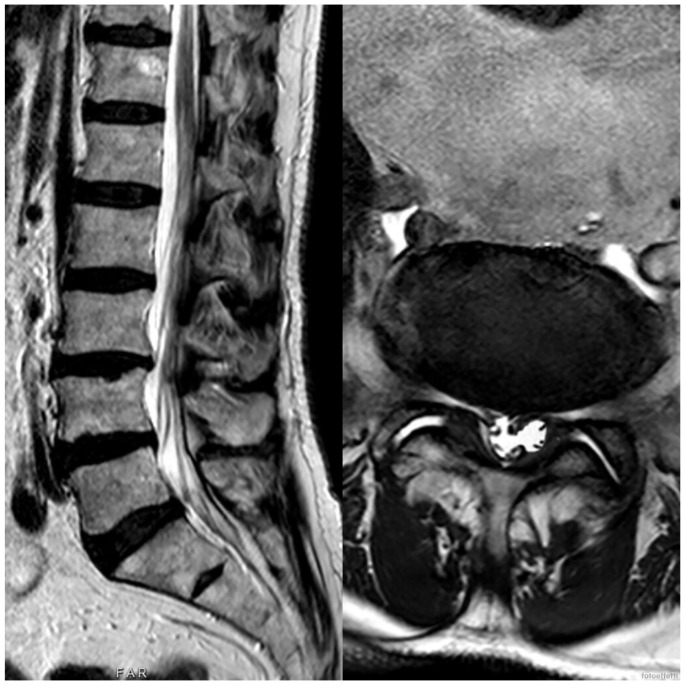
Preoperative MRI of the lumbo-sacral spine of the second patient; here, we can observe the left L4/L5 synovial cyst.
